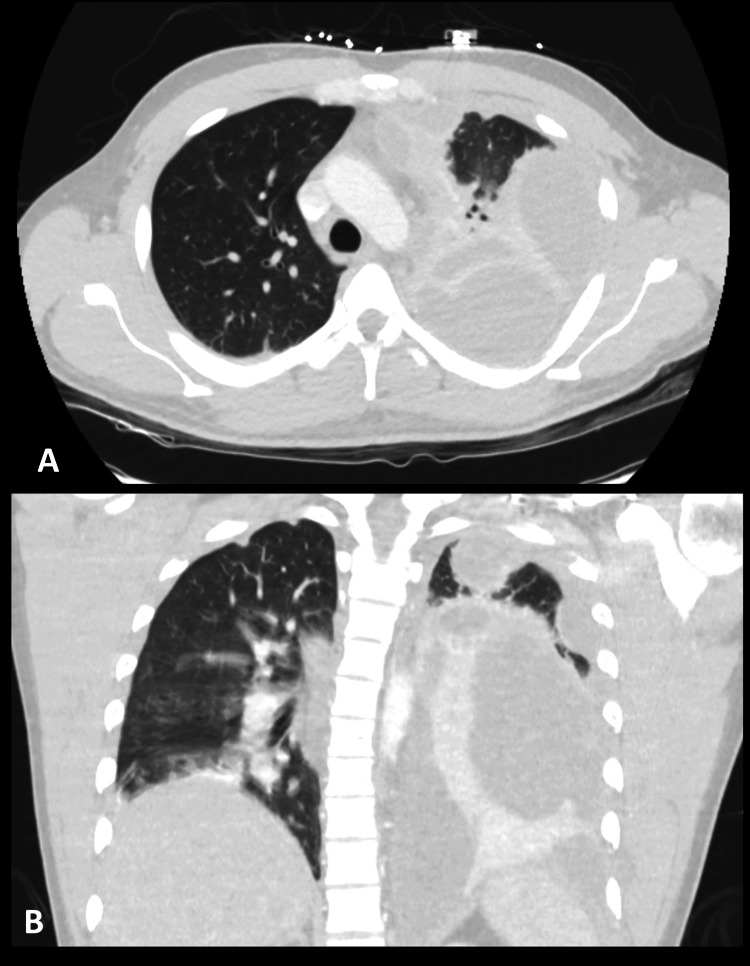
(A) CT angiography of the chest with contrast showing loculated left-sided collections with associated pleural thickening. (B) CT angiography of the chest with contrast in a coronal plane showing loculated left-sided collections with associated pleural thickening. Complete atelectasis of the left lower lobe and lingula can be noted.
